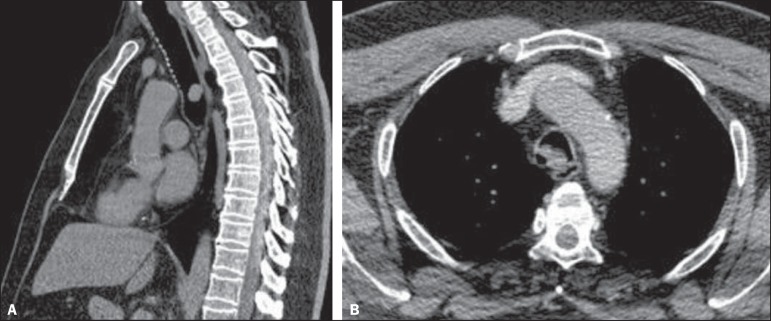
A: Sagittal CT scan (ROI: 51 HU), without contrast, showing an
